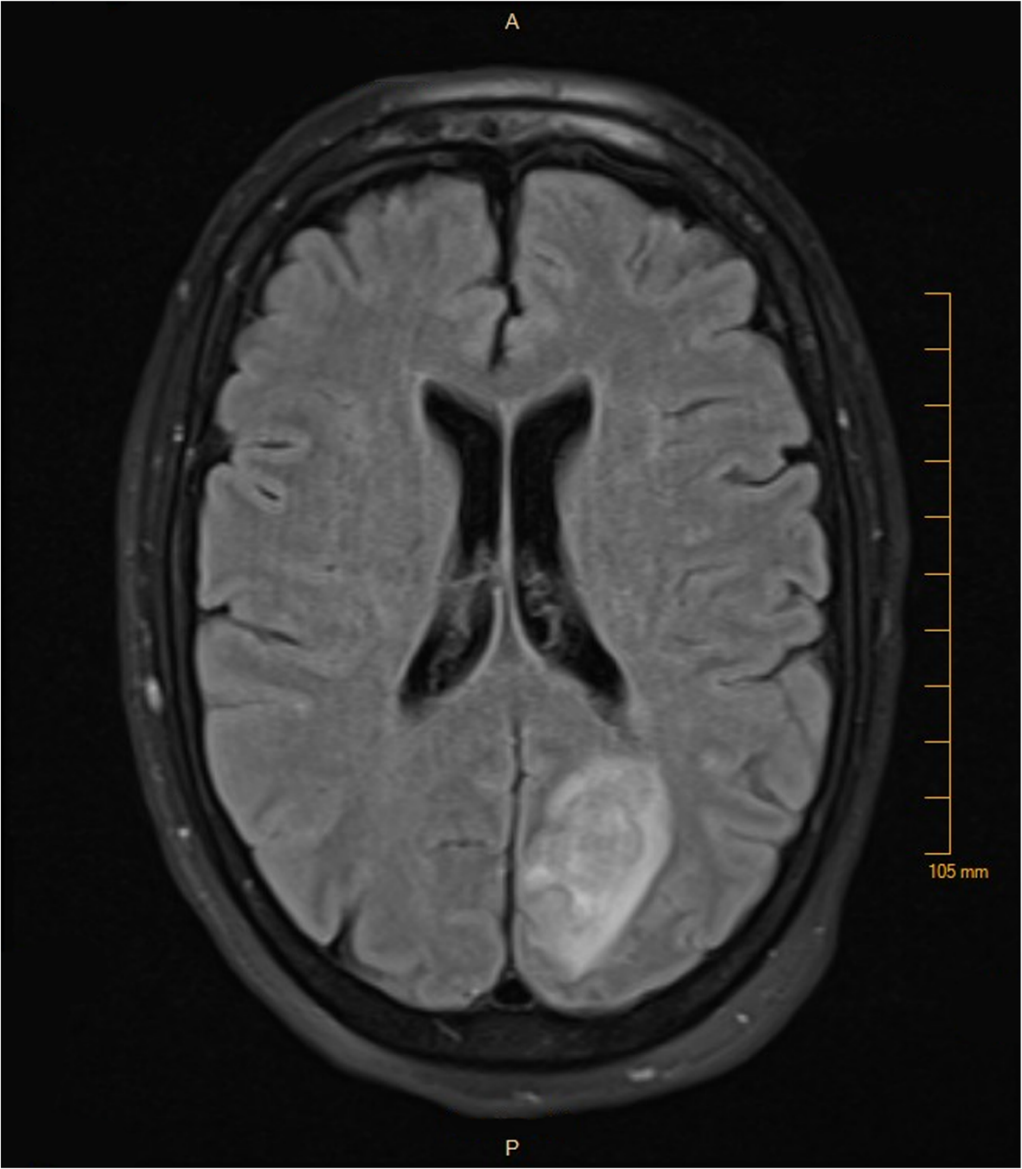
MRI of the patient’s brain on hospital day 3, revealing a left-sided parieto-occipital hemorrhagic infarct
